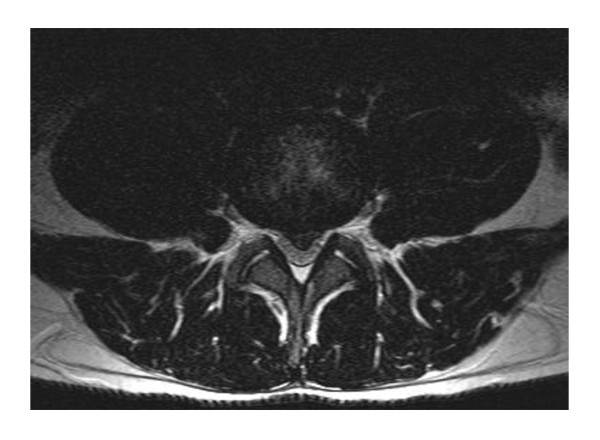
The corresponding axial T2-weighted MRI image at L4/L5 demonstrates the centrally herniated disk material narrowing the spinal canal.
